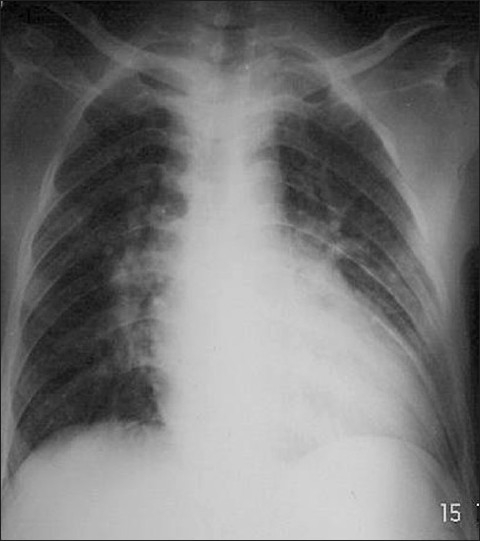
Chest X-ray - Cardiomegaly with pulmonary plethora with a narrow pedicle (case 1)
